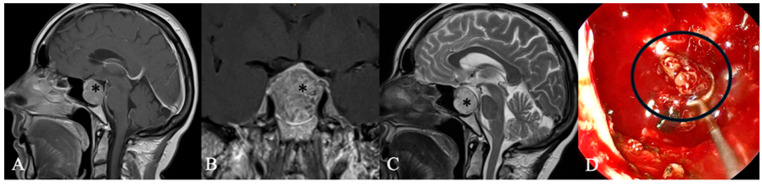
A 59-year-old female patient with the diagnosis of an extradural chordoma. (A) Sagittal and (B) coronal contrast-enhanced MRI showing a heterogenous contrast-enhancing mass (*) intrasellar. (C) T2 Imaging with heterogenous high signal. Bony destruction of the clivus is common. (D) Intraoperative findings revealed fibrous intrasellar tissue, more adherent to the cavernous sinus wall than PitNETs, with no pseudocapsule. While tumor resection was feasible, en bloc removal was not possible. The patient returned to work six months after diagnosis, with no recurrence observed 15 months post-surgery.
